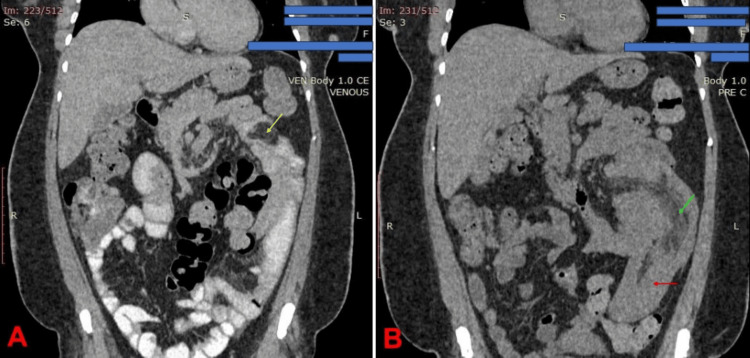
CT image of the abdomen in coronal view (A) Contrast image showing a hypodense mass appearing as a filling defect within the ileum (yellow arrow). (B) Pre-contrast image showing the mesenteric fat associated with the intussusceptum (green arrow), and the wall of the intussuscipiens (red arrow).
